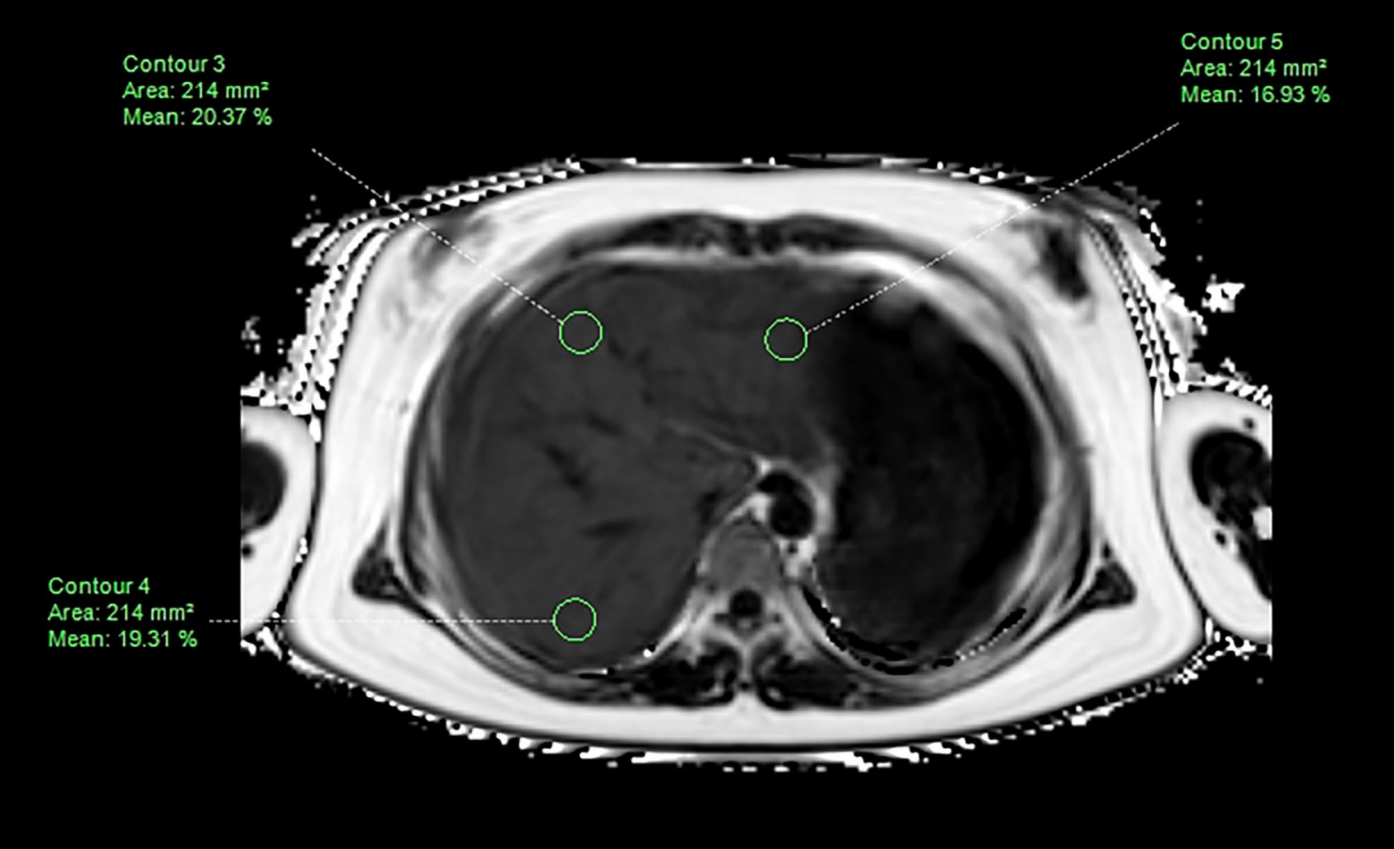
Liver fat content measurement with chemical shift-encoded magnetic resonance imaging
